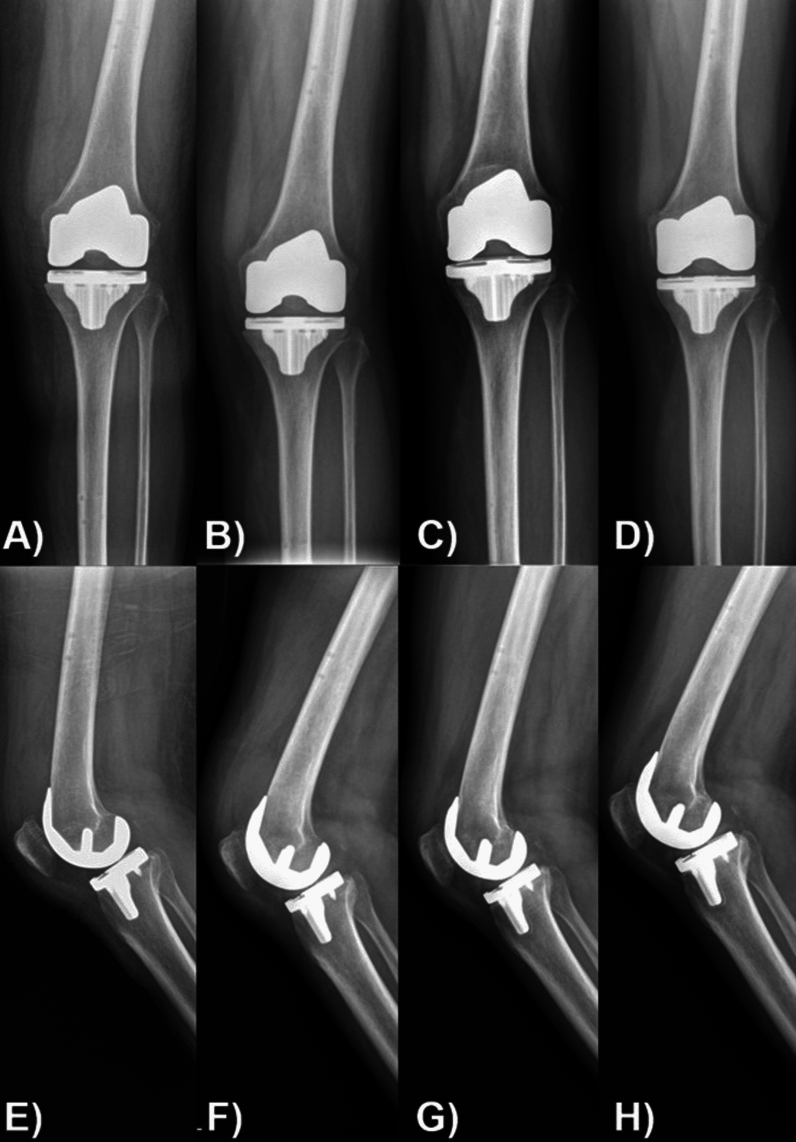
Radiographic images of a 64-year-old female patient with osteopenia and overweight (BMI: 27.04 kg/m^2^) undergoing left cementless total knee arthroplasty demonstrate progressive posterolateral subsidence of the tibial baseplate over time, particularly when comparing the serial anteroposterior (A–D) and lateral (E–H) views. A, E Immediate postoperative X-rays; B, F 1 month postoperative; C, G 6 months postoperative; and D, H 1 year postoperative
